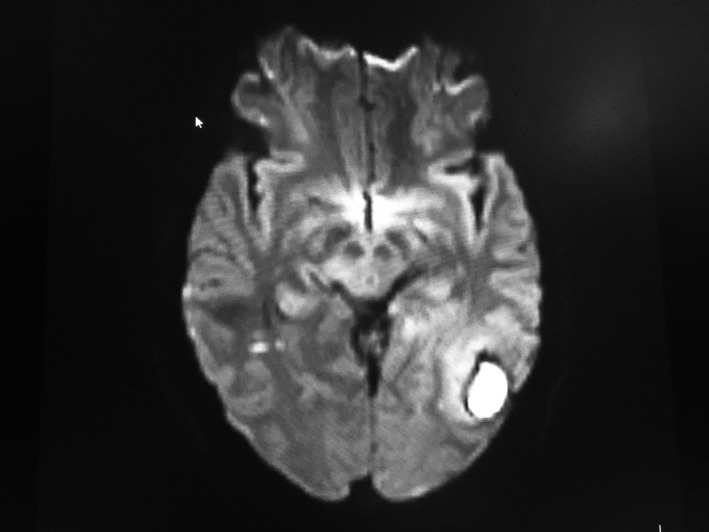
MRI brain showing intracerebral hematoma in the left parieto‐occipital region
